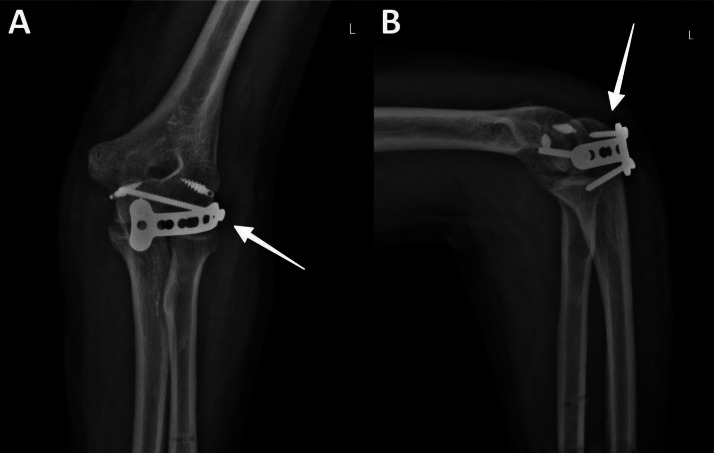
Postoperative radiographic assessment. (A) Anteroposterior X-ray view showing reduced elbow joint with T-plate fixation (white arrow) spanning the joint. The alignment of the ulnohumeral and radiohumeral joints is preserved. (B) Lateral X-ray view demonstrating congruent reduction of the ulnohumeral articulation with T-plate stabilization (white arrow) and appropriate screw placement in the olecranon and distal humerus. Note the restoration of the ulnohumeral line passing through the middle of the joint and the intersection of the radiocapitellar line with the capitellum, indicating proper reduction.
